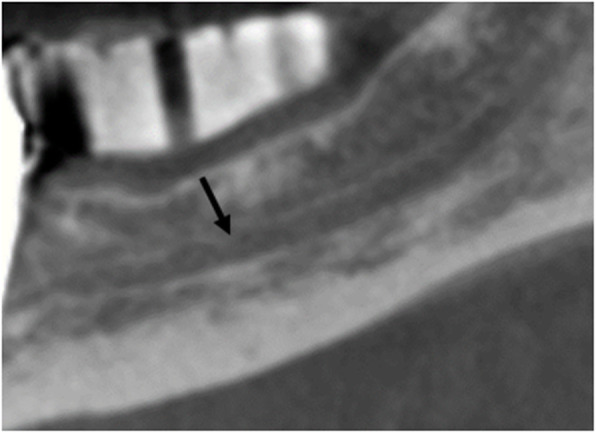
CBCT: region 36 canal roof visible
